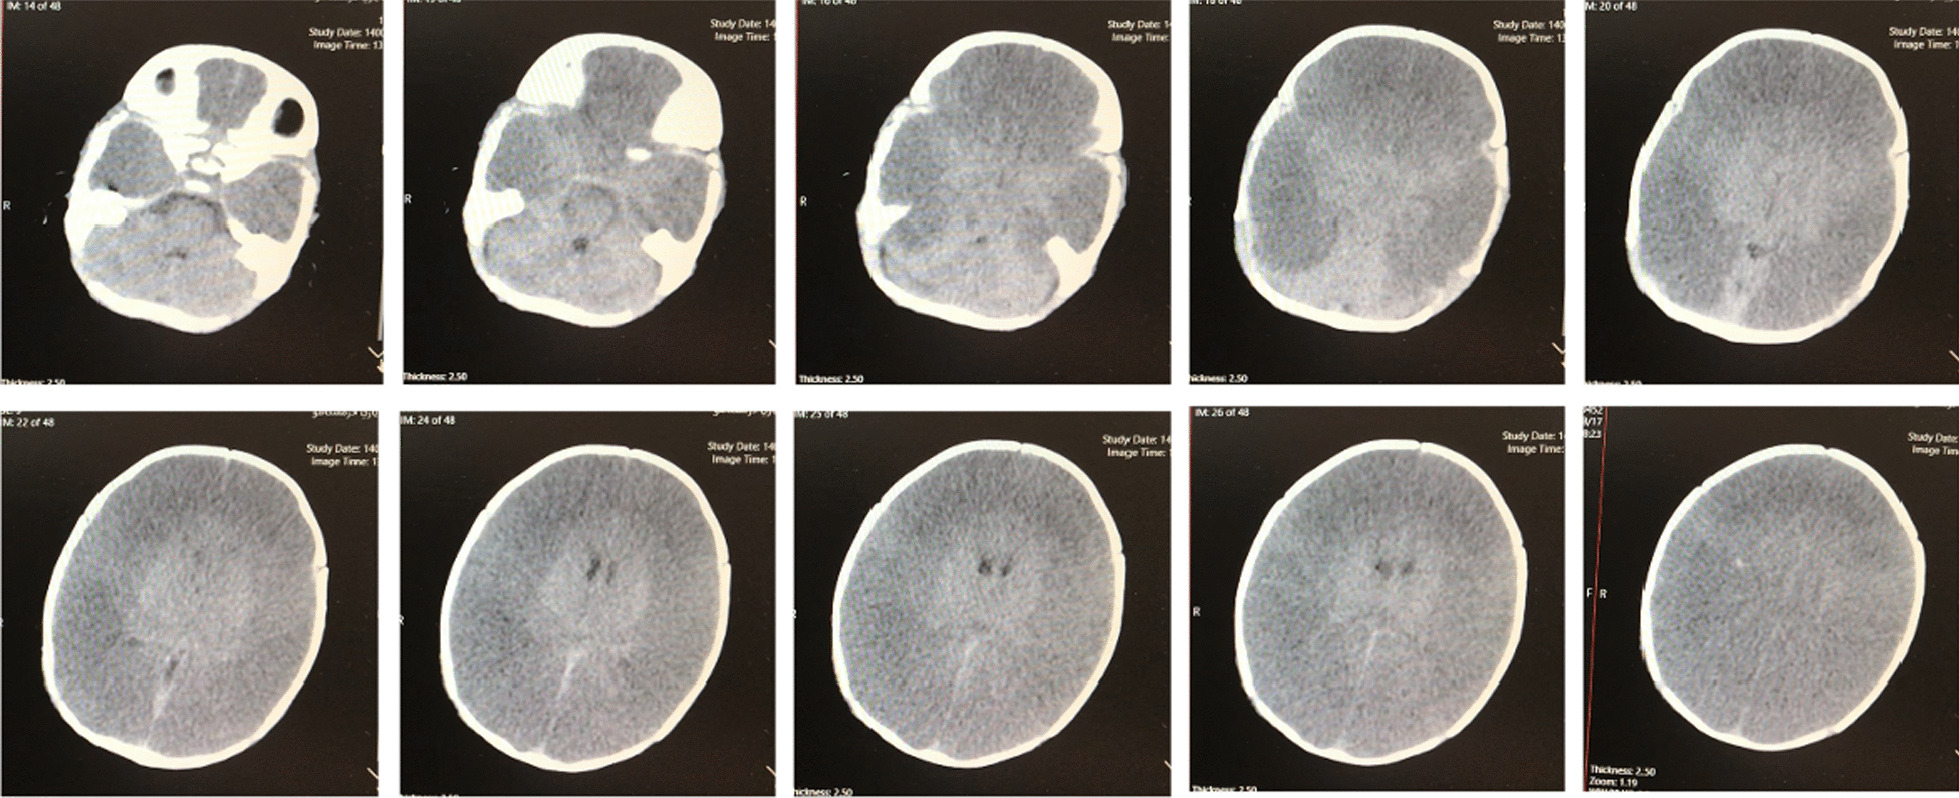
Brain computed tomography scan indicating evidence of severe diffuse cerebral edema, including brain ventricle effacement and fading of the brain sulci
